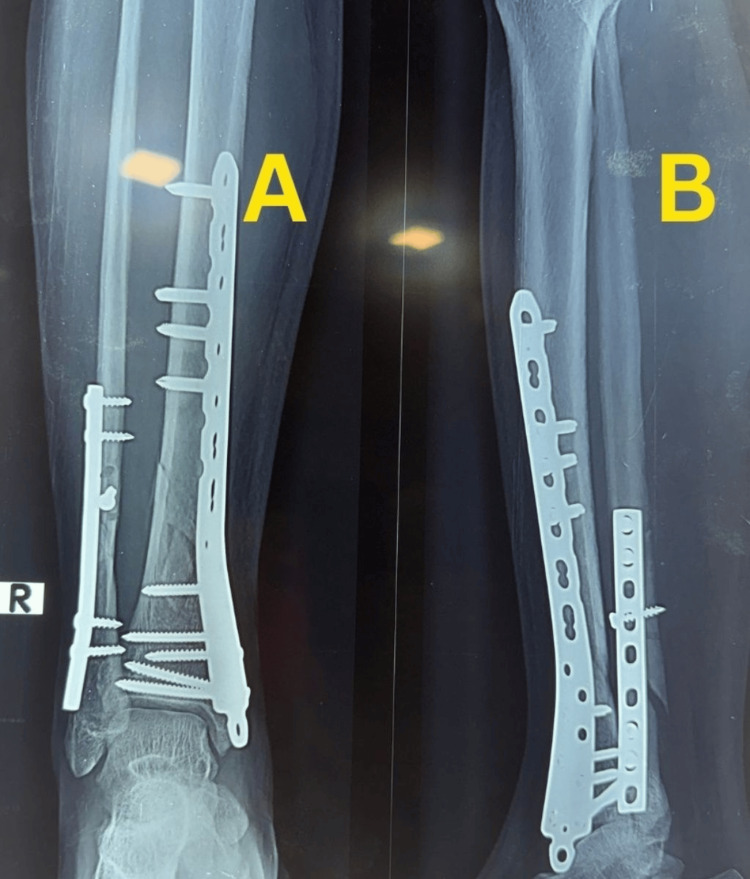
Early follow-up radiographs of Case 1. (A) Anteroposterior view demonstrating maintained alignment with early callus formation at the fracture site. (B) Lateral view showing progression of healing without loss of reduction.
